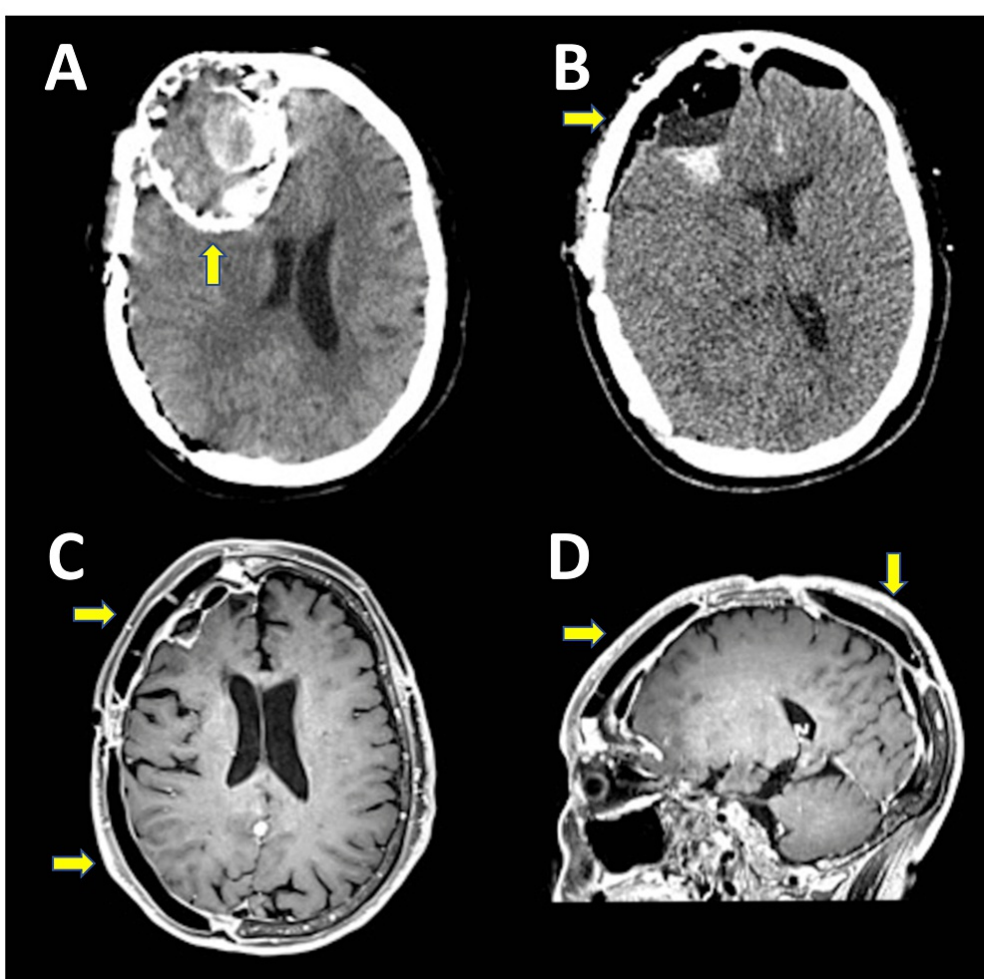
Preoperative and postoperative CT scans and MRI images. (A) An axial section in a CT scan shows a frontal tumor with soft tissue density and intracranial extension (yellow arrow). (B) An axial section with postoperative changes due to craniectomy and right frontal cranioplasty (yellow arrow). (C) An axial section in the MRI shows postoperative changes secondary to the resection of right frontal and parietal lesions (yellow arrows). (D) Postoperative MRI in a sagittal section showing (yellow arrows). CT: computed tomography.
